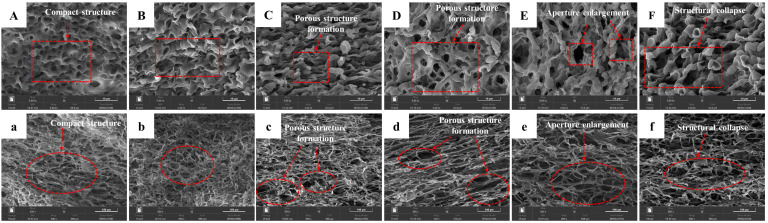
Microstructure of dried *C. cibarius* pileus under different ultrasound power pretreatment conditions: (A) control, (B) 100 W, (C) 200 W, (D) 300 W, (E) 400 W, and (F) 500 W. Microstructure of the longitudinal section of dried *C. cibarius* stipe under different ultrasonic power pretreatment conditions: (a) control, (b) 100 W, (c) 200 W, (d) 300 W, (e) 400 W, and (f) 500 W.
